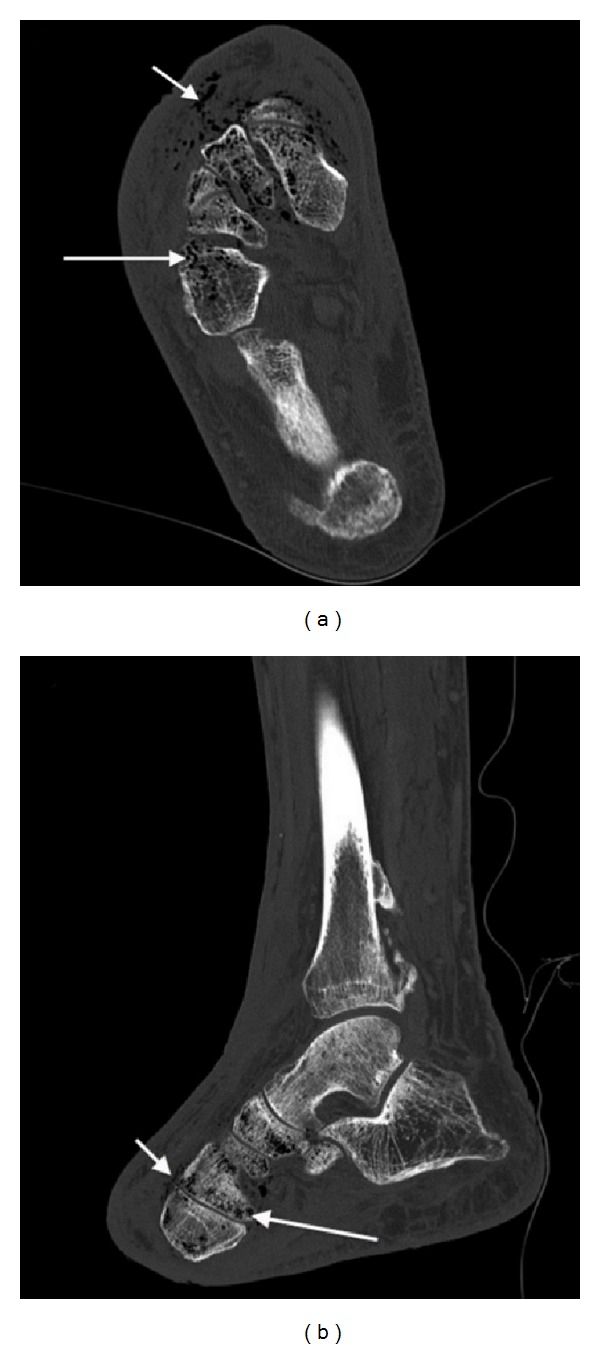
Computed tomography demonstrates gas within the midtarsal bones (long arrow, (a) and (b)) and head of talus and gas in the soft tissues surrounding the midfoot (short arrow, (a) and (b)).
